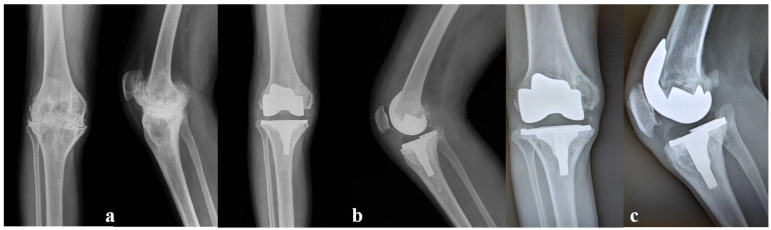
A 39-year-old patient with severe arthropathy of his left knee—Pettersson score 12 (a), undergoing a posterior stabilized TKA. X-rays at 1 year (b) and 16 years (c) after surgery. The implant was still in place without significant osteolysis or radiolucency. The patient was still satisfied, and his joint was well functioning.
